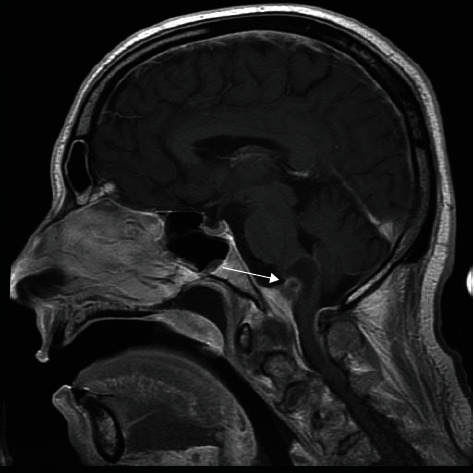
Preoperative MR brain T1-weighted sagittal image demonstrates a cystic mass arising at the C1-C2 level extending cephalad traversing the foramen magnum. The mass insinuates between the intradural segments of the vertebral arteries bilaterally causing severe compression at the cervicomedullary junction with the associated cystic area in the caudal medulla.
